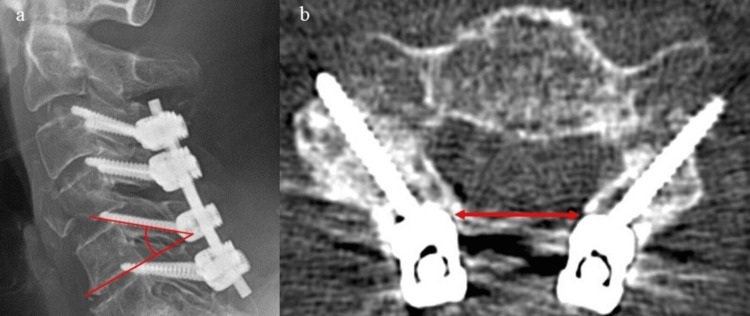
Screw angle and distance between screw heads a: Screw angle (the angle between the extension of the inferior vertebral margin of each vertebra and the screw). If the angles of the left and right screws were different, the average angle was used. b: Distance between screw heads was measured as the medial distance between the left and right screw heads. Postoperative CT axial images were used for evaluation.
